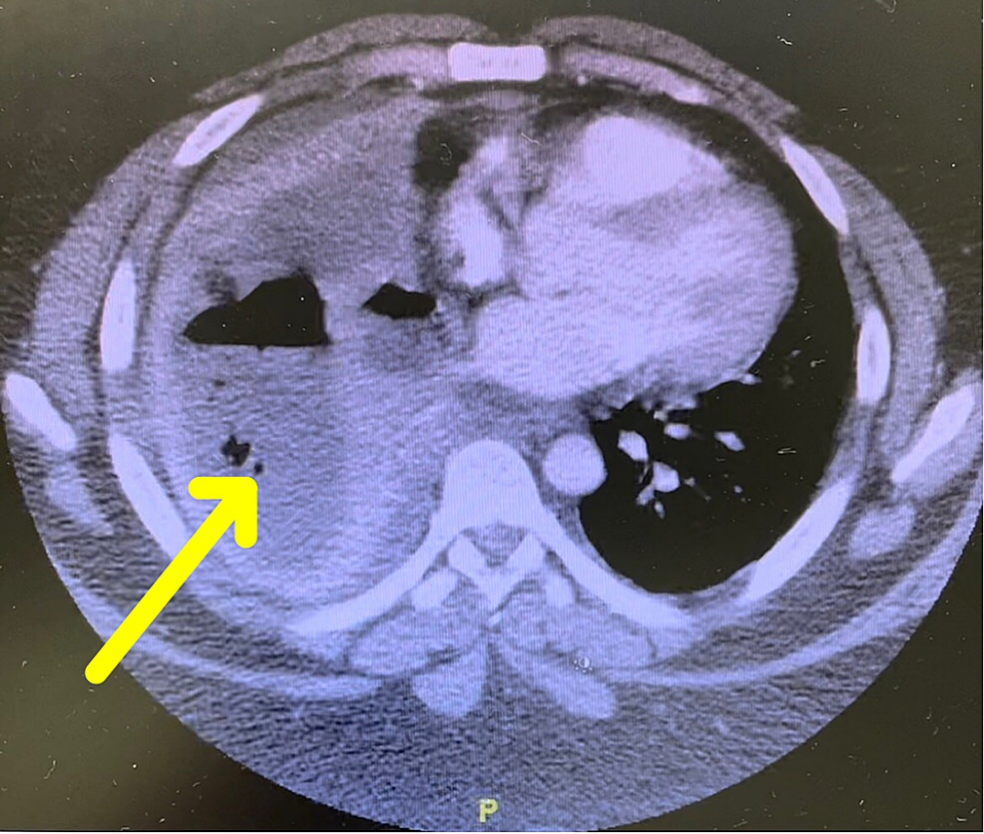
Computed tomography (CT) of the chest from the initial day of presentation revealing a 12.2 cm x 12.1 cm loculated gas and fluid collection within the right lower lobe concerning for pulmonary abscess vs empyema (yellow arrow)
